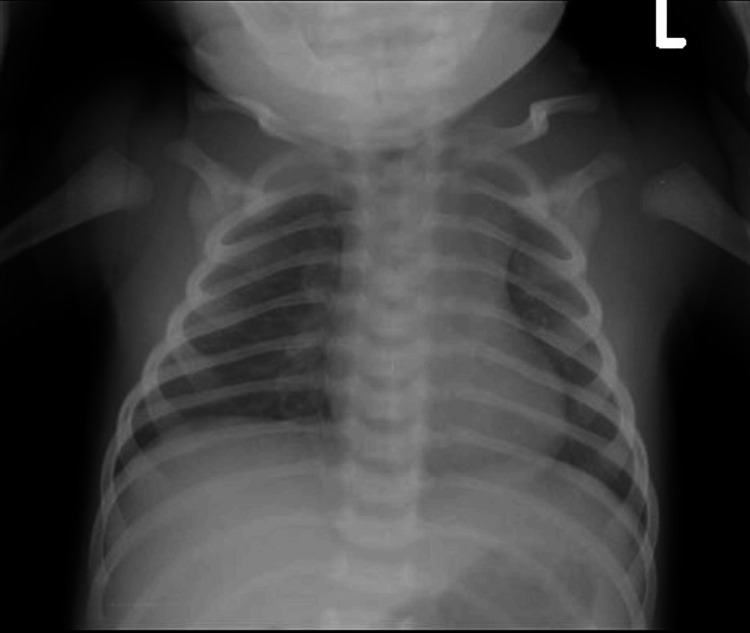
Neonatal chest radiograph Chest radiograph of a seven-day-old neonate demonstrating well-expanded lungs and no evidence of focal airspace opacities, pleural effusion, or pneumothorax.
